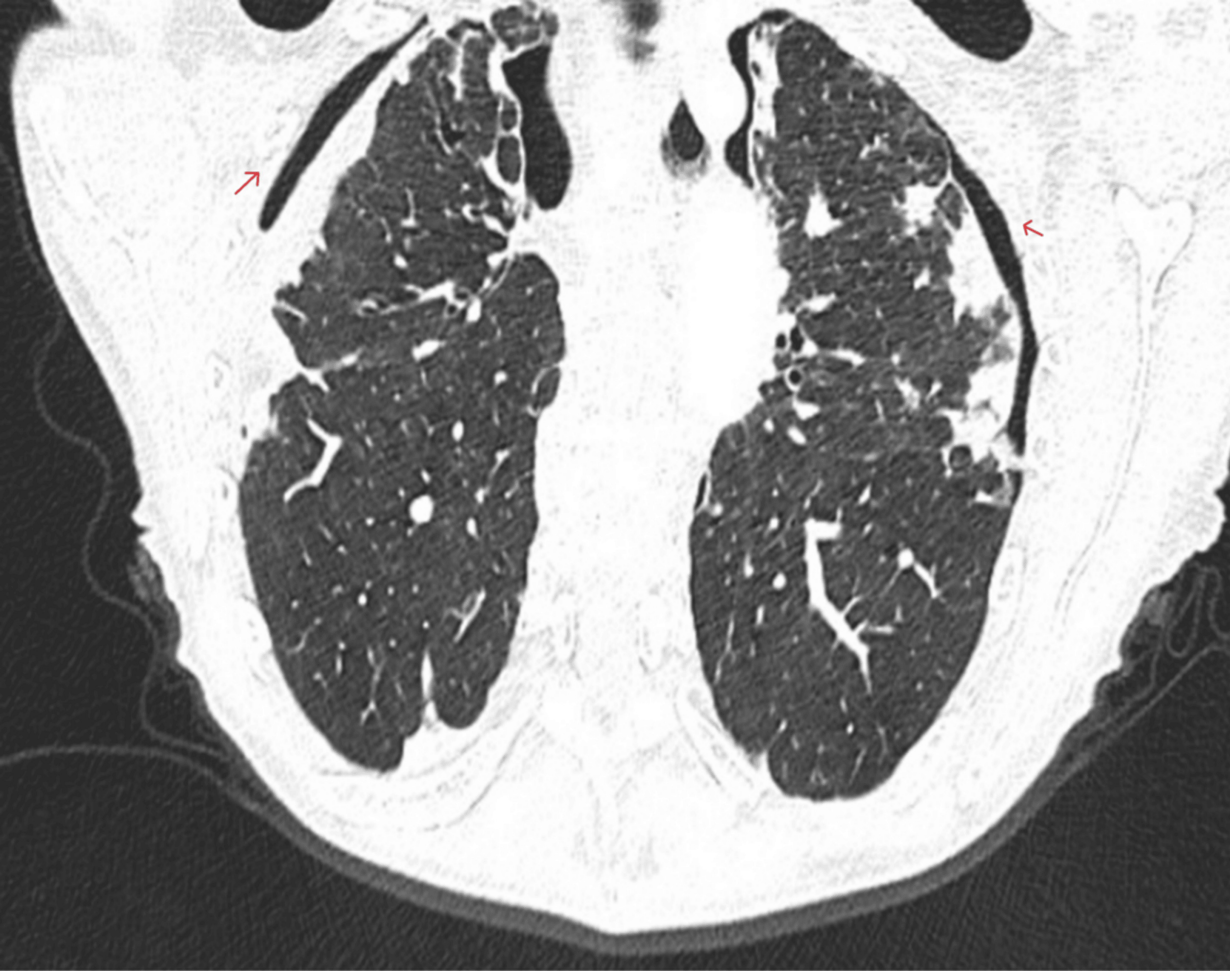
CT pulmonary angiogram showing apical pneumothoraces (red arrows), which are persistent and stable compared to the previous CT thorax with contrast.
